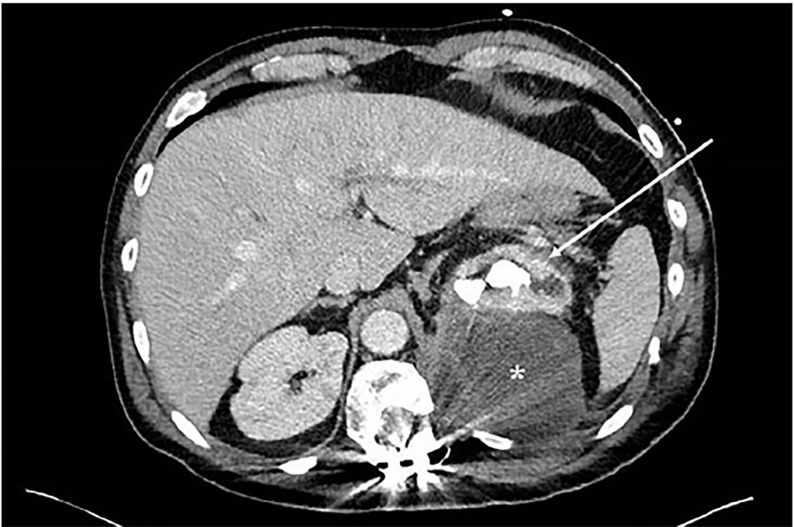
Computed tomography, axial image, demonstrating large fluid collection (*) centered over the left psoas. The left kidney is displaced and demonstrates a staghorn calculus with cystic changes contiguous with the fluid collection (arrow). There is artifact from spinal fusion hardware.
